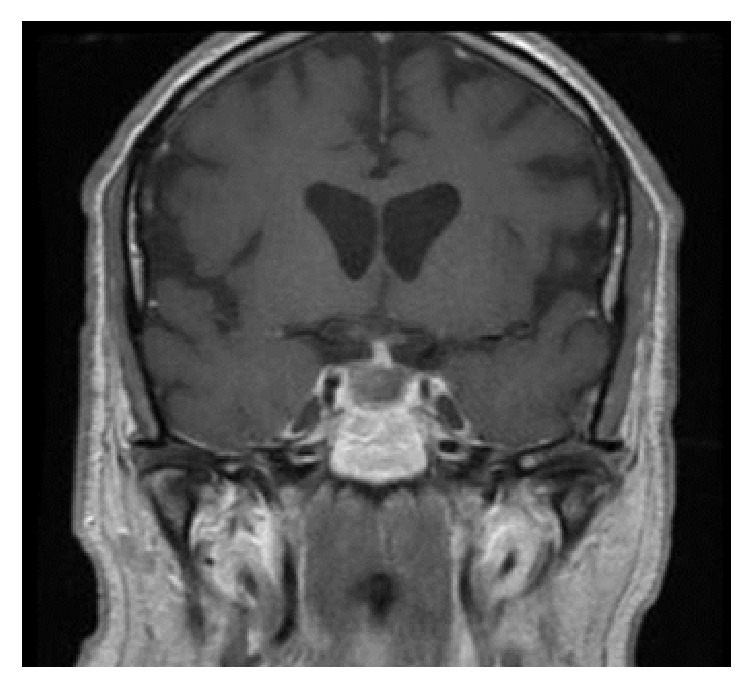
MRI (T1 weighted with contrast) one day after the surgery shows interval resection of the previously seen sellar mass/hemorrhage and resolution of mass effect on the optic chiasm.
